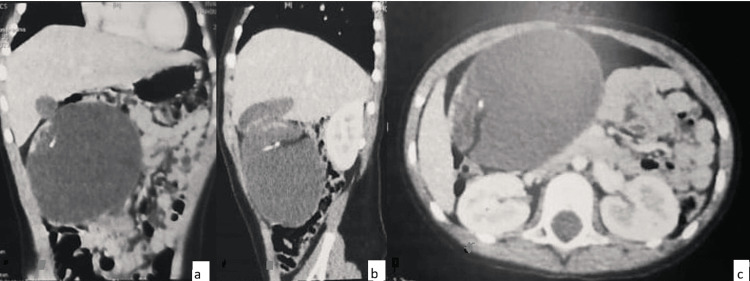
CT scan showing coronal (a), sagittal (b), and axial (c) sections revealing a cystic mass on the left side measuring 90x76x98 cm, the presence of a fatty component, calcium, and a few thin superolateral right septa
